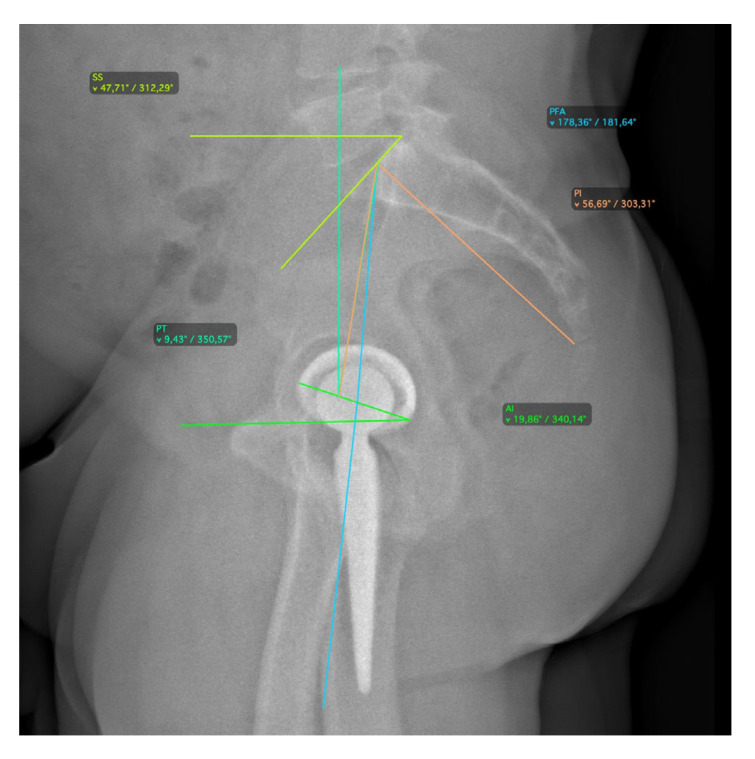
Radiographic measurements for spinopelvic parameters in standing position.
